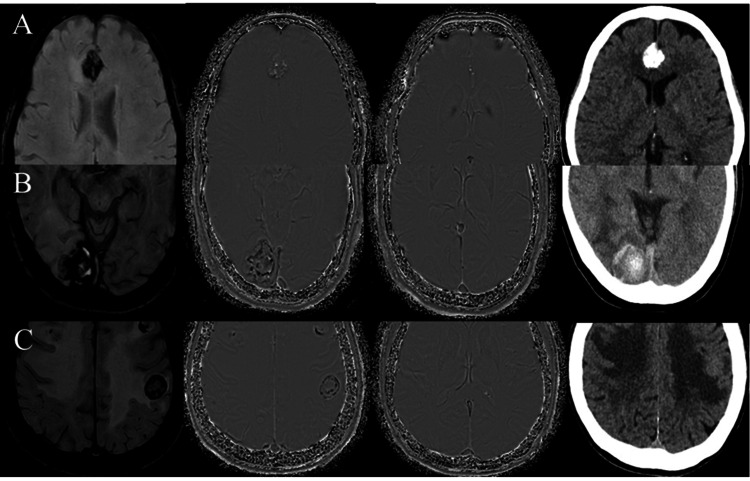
ICVs as the reference venous structure on SWI phase image for differentiating intratumoral calcification (A), gross hemorrhage (B), and microbleed (C) compared with CT CV: internal cerebral vein; CT: computed tomography; SWI: susceptibility-weighted imaging
